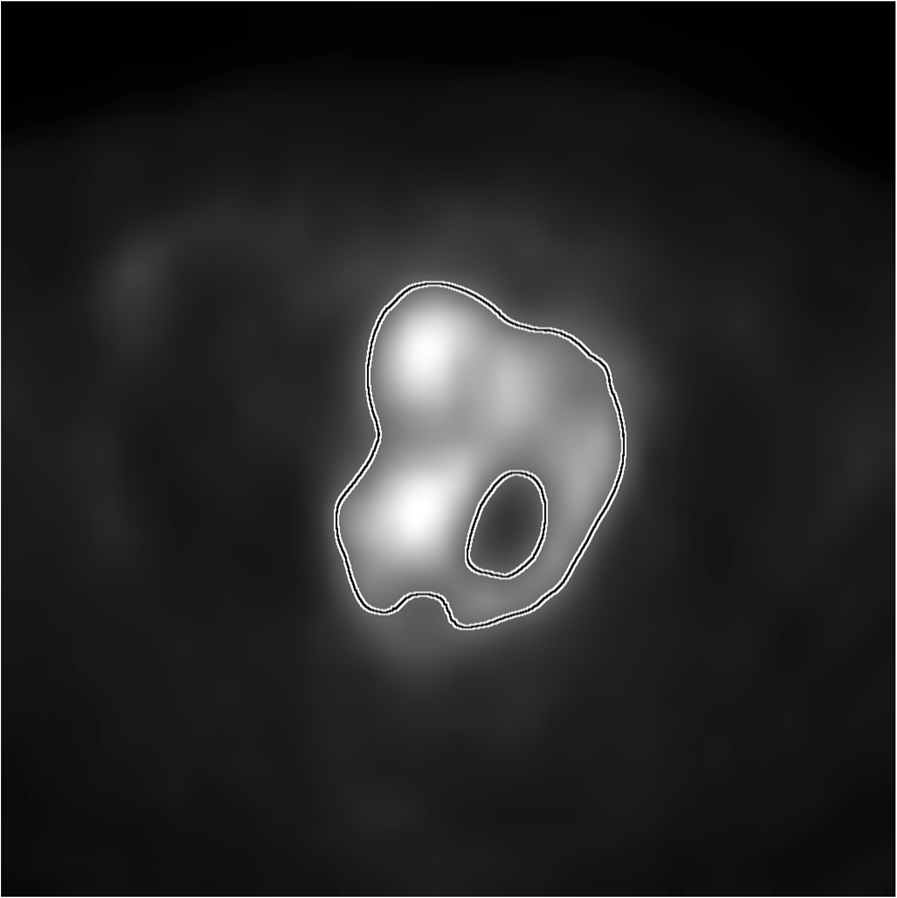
Shown is an example of a cervical carcinoma imaged via FDG-PET. The black and white contour approximately indicates the defined tumor boundary. Within, there are clear variations in the grayscale intensity. It is these variations researchers seek to objectively quantify such that inter-patient comparisons of *intra*-tumor uptake heterogeneity can be made. The image edges correspond to 20 cm within the patient.
